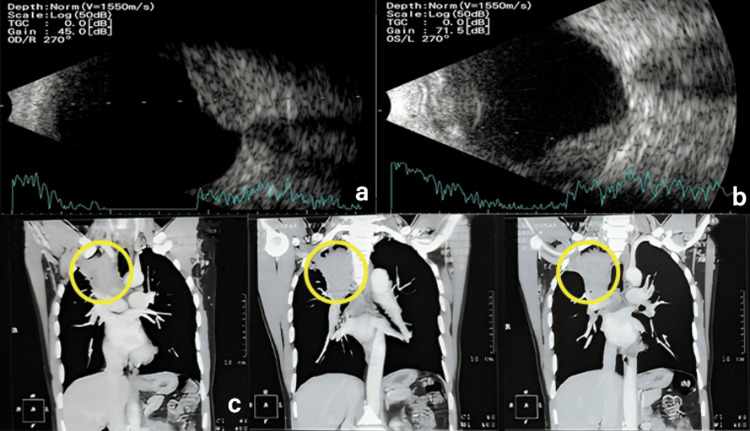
At presentation. (a, b) B-scan ultrasound showing a diffuse homogenous mass lesion involving the retinochoroidal complex with subretinal fluid and medium internal reflectivity OU. (c) Coronal section of CT chest showing heterogeneously enhancing soft tissue density mass lesion (yellow circles) seen in the apical segment of right upper lobe extending up to the right hilar region. OS: oculus sinister; OD: oculus dexter; OU: oculus uterque.
